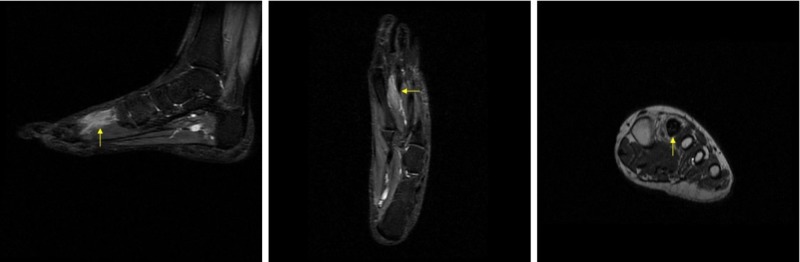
Pre-operative magnetic resonance imaging (MRI) showing cement in second metatarsal. From left to right: sagittal STIR MRI; coronal STIR MRI; axial T2 MRI.
